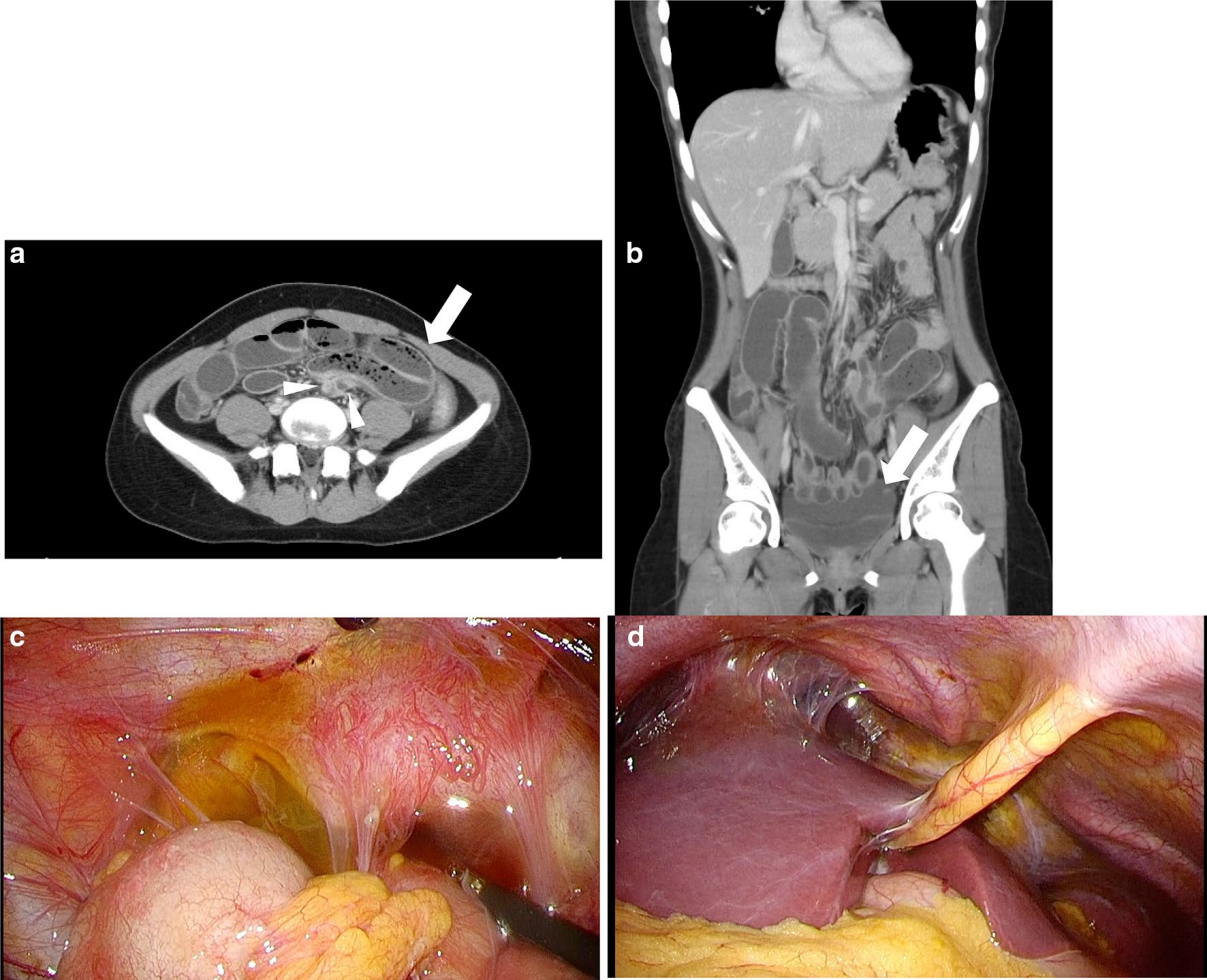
Abdominal CT and intraoperative findings. a arrow: Closed loop formed and dilated small intestine, arrowheads: Collapsed small intestine on the oral and anal sides of the closed loop, b ascites in the pelvis, c ascites in the pelvis and adhesion of the uterine appendages, and d adhesions that are translucent and considered to be in the active stage of chlamydial infection on the liver surface
